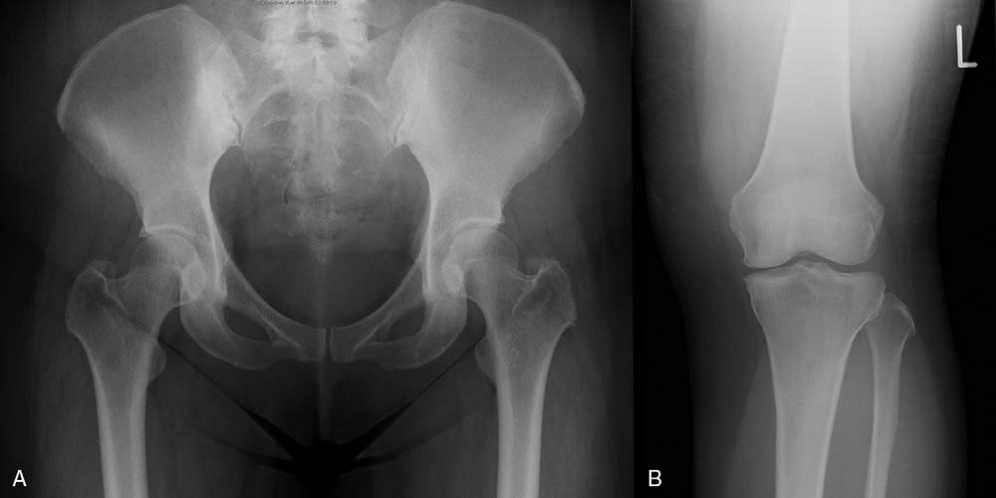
X-rays of the pelvis (A) and left knee (B) showing osteophytes and joint space narrowing, consistent with osteoarthritis.
